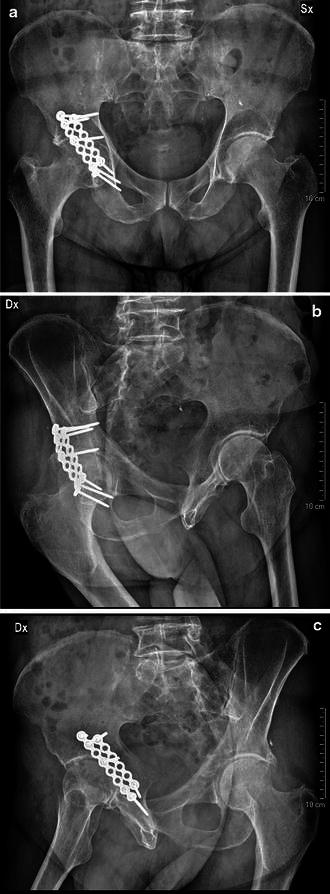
Post-operative X-rays: a AP and b, c oblique Judet views (same patient as in Fig. 1)
